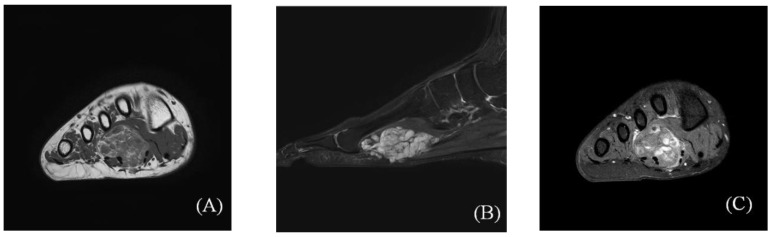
Preoperative magnetic resonance imaging (MRI) scans showing a 5.6 × 2.8 × 2.5 cm multilobulated soft tissue mass in the flexor digitorum brevis muscle, which penetrates the plantar fascia and spreads to the subcutaneous layer. (A) Axial T1-weighted images; (B) sagittal T2-weighted images; (C) axial gadolinium T1-weighted image.
